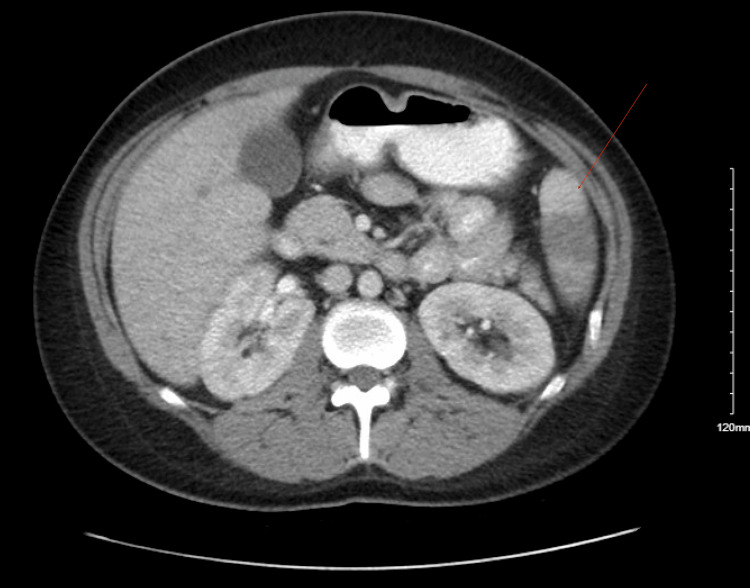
CT of the abdomen performed in the emergency department demonstrating multiple wedge-shaped non-enhancing regions off the radius for pain consistent with infarcts of the spleen.
